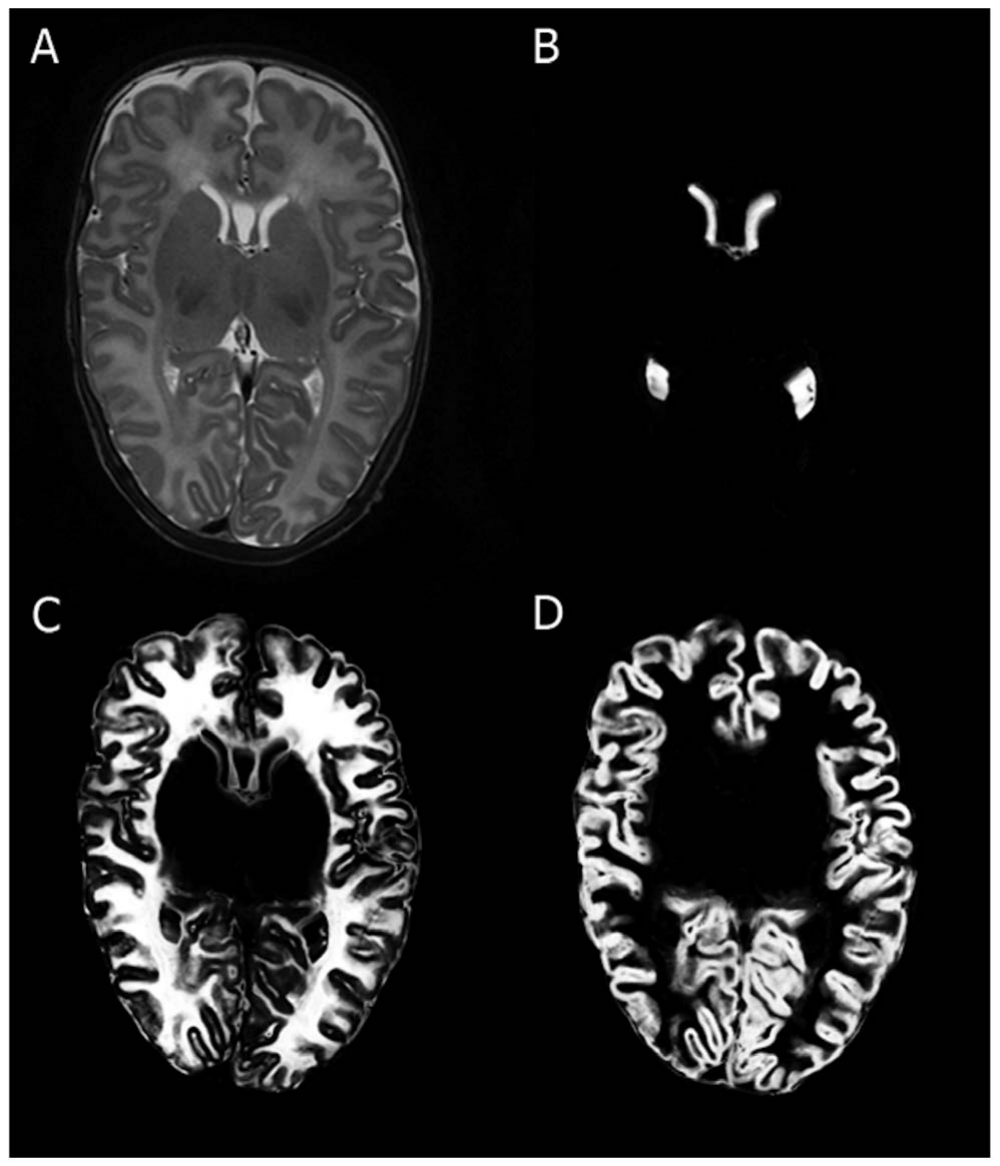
Representative example of a T2-weighted scan (fig. 2A) with segmentations of cerebral ventricles (fig. 2B); unmyelinated white matter (fig. 2C); and cortical grey matter (fig. 2D).
